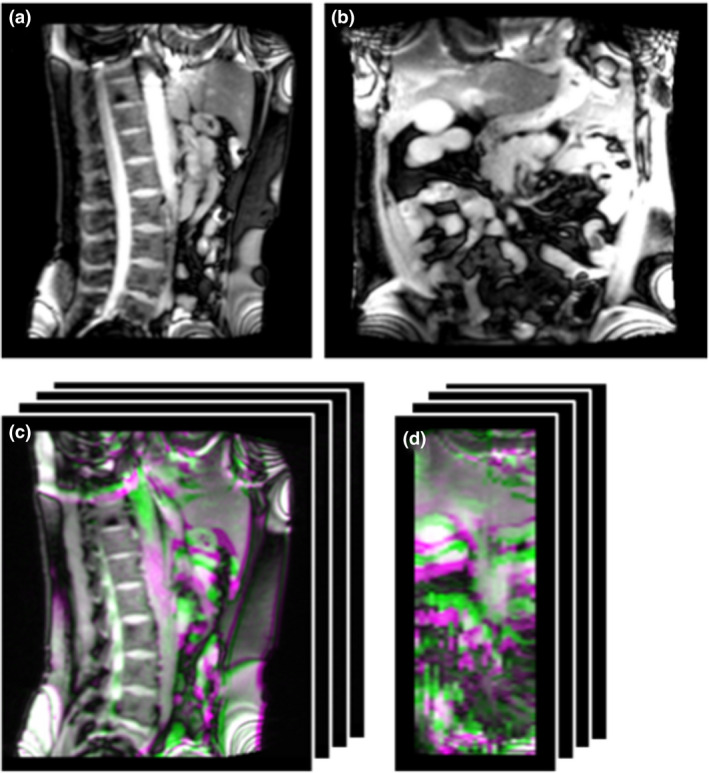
Example of acquired data (P02): sagittal (a) and coronal (b) cine‐MRI frames; sagittal (c) and coronal (d) view of the overlay between the end‐exhale (green) and end‐inhale (violet) phases of the 4DMRI.
